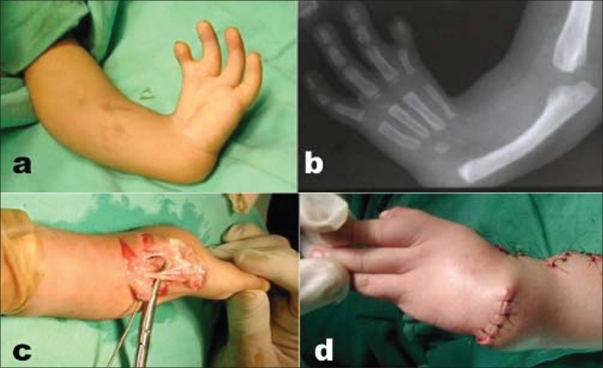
(Case no. 9) (a) clinical photograph showing grade IV radial club hand left side (b) X-ray forearm (anteroposterior view) with elbow, wrist and hand showing radial club hand grade IV (c) peroperative photograph showing the abnormal median nerve with its cutaneous branches (d) clinical photograph showing correction attained immediate post operatively
